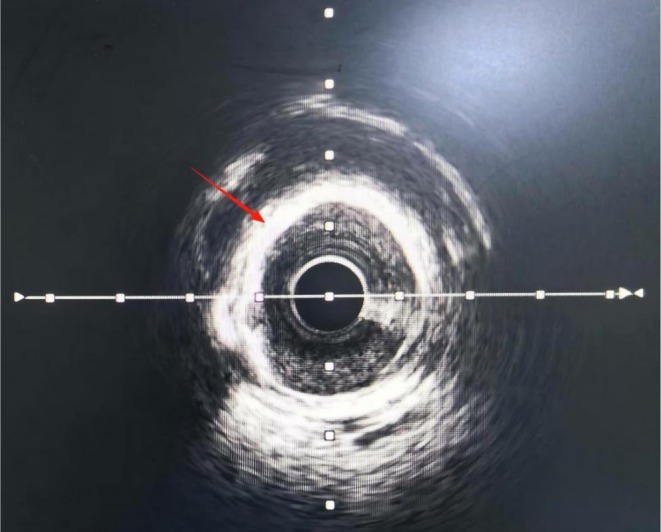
Intravascular ultrasound imaging of the coronary artery showing 270°–360° circumferential calcification.
